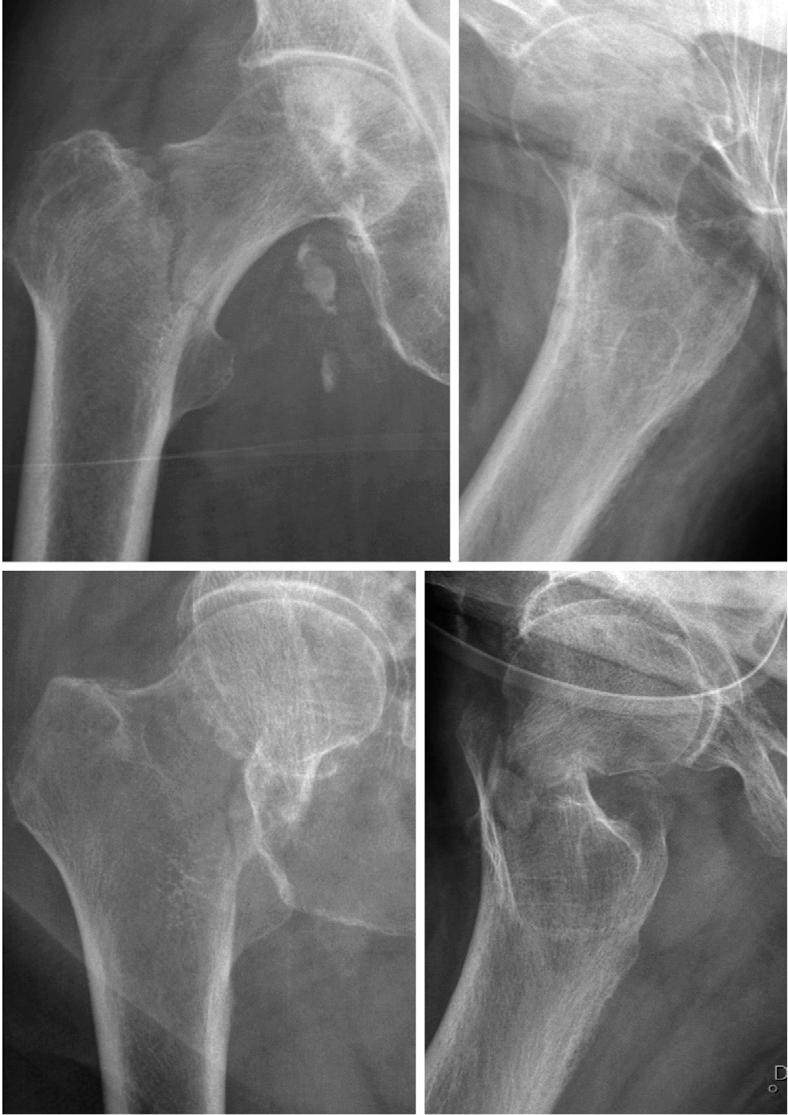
Antero-posterior and lateral radiograph of a true bFNF (top) and a displaced transcervical FNF (bottom). The lateral radiograph reveals the femoral neck attached to the proximal fragment (top) and distal fragment (bottom).
